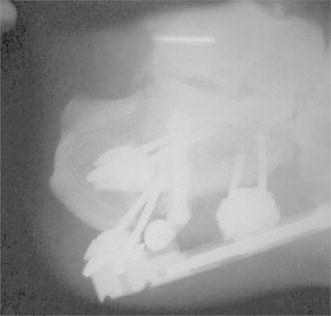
Postoperative radiograph on lateral view
